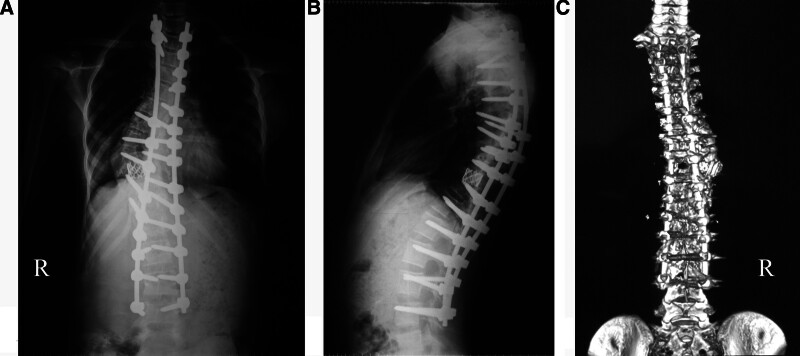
Posterior vertebral column resection (PVCR) at T10 level to correct the deformity, spinal shortening, deformity correction, T2-L4 fixation with pedicle screws and rods, and spinal fusion with titanium mesh and bone grafts. (A) A postoperative AP X-ray shows a Cobb angle of the coronal main curve of 22°, RSH of 0 cm, and C7-CSVL of 1.5 cm. (B) On a lateral X-ray, the Cobb angle is 34°, and the SVA is 2.4 cm. (C) Postoperative 3-dimensional CT image reconstruction showing posterior spine and implants. C7-CSVL = C7-central sacral vertical line, CT = computed tomography, PVCR = posterior vertebral column resection, RSH = radiographic shoulder height, SVA = sagittal vertical axis.
